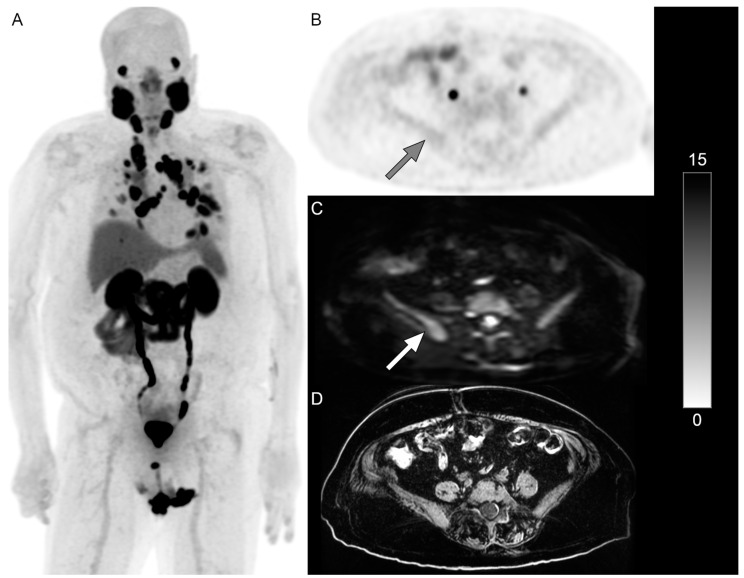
PET/MRI in a 78-year-old man with mCRPC with extensive mediastinal and supraclavicular nodal metastases ((A), whole-body ^18^F-DCFPyL PSMA PET maximum-intensity projection image). Only minimal PSMA activity was identified in the pelvic bones ((B), gray arrow); however, osseous metastases were identified on b = 800 DWI ((C), white arrow). A T1-weighted image (D) is also shown. The scale bar of PET activity (SUVs) is on the right. RLT was planned, but he died a month later prior to receiving RLT.
